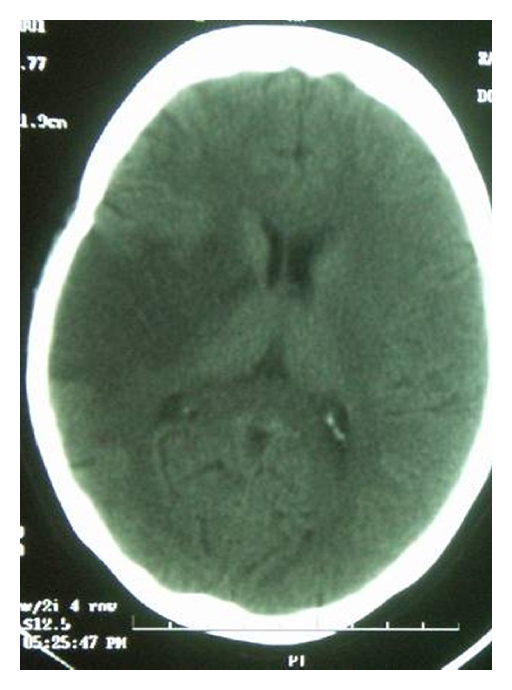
Plain CT scan brain showing a massive right MCA territory infarct during admission.
